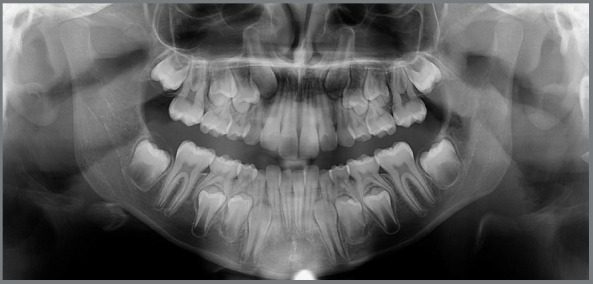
Panoramic radiograph taken at 8 years of age.
